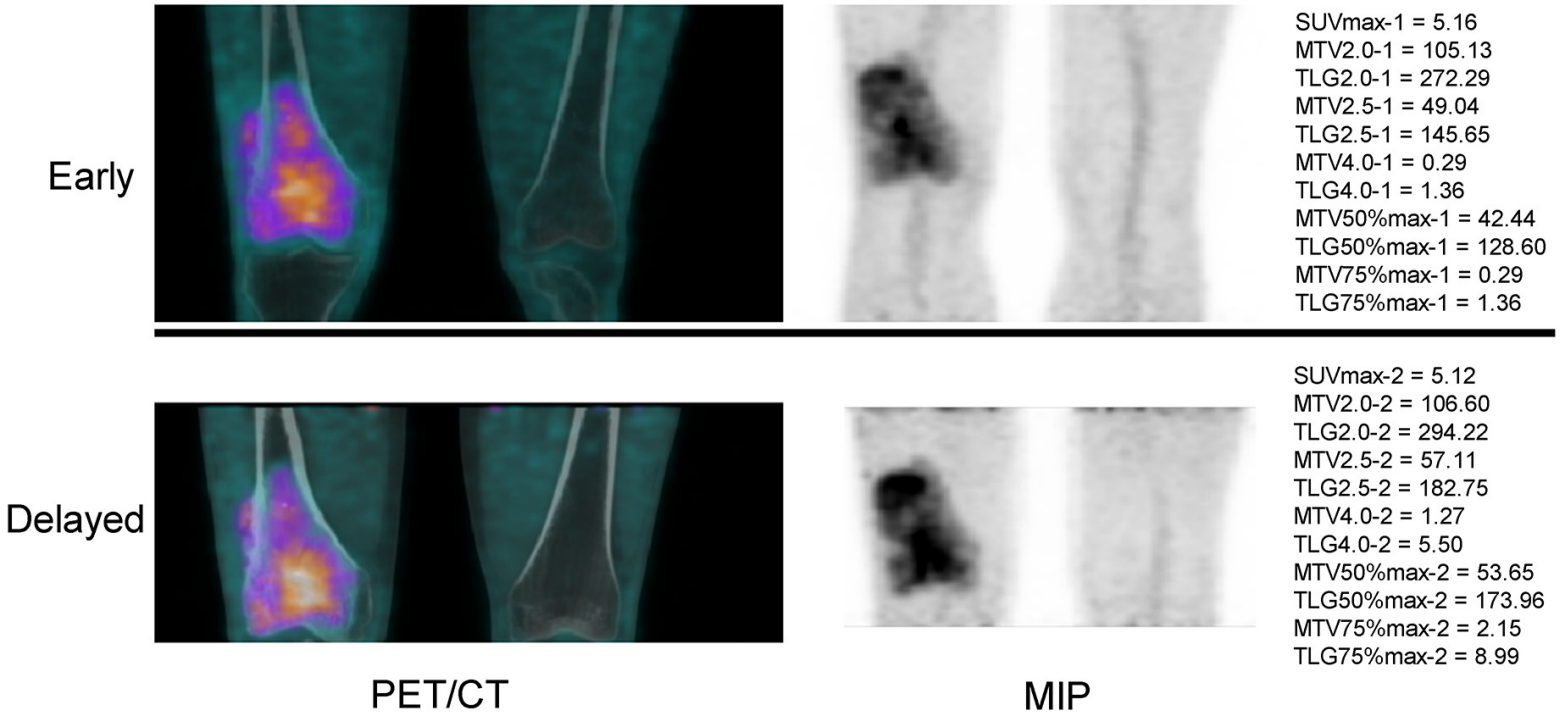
^18^F-FDG PET/CT imaging of a 47-year-old male who was diagnosed of osteosarcoma after surgery MIP, maximal intensity projection.
